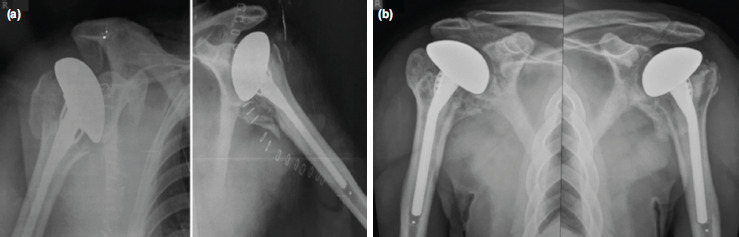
(a) Immediate post-operative and (b) two years' follow-up radiographs of both shoulders showing positions of the implants without complications.
